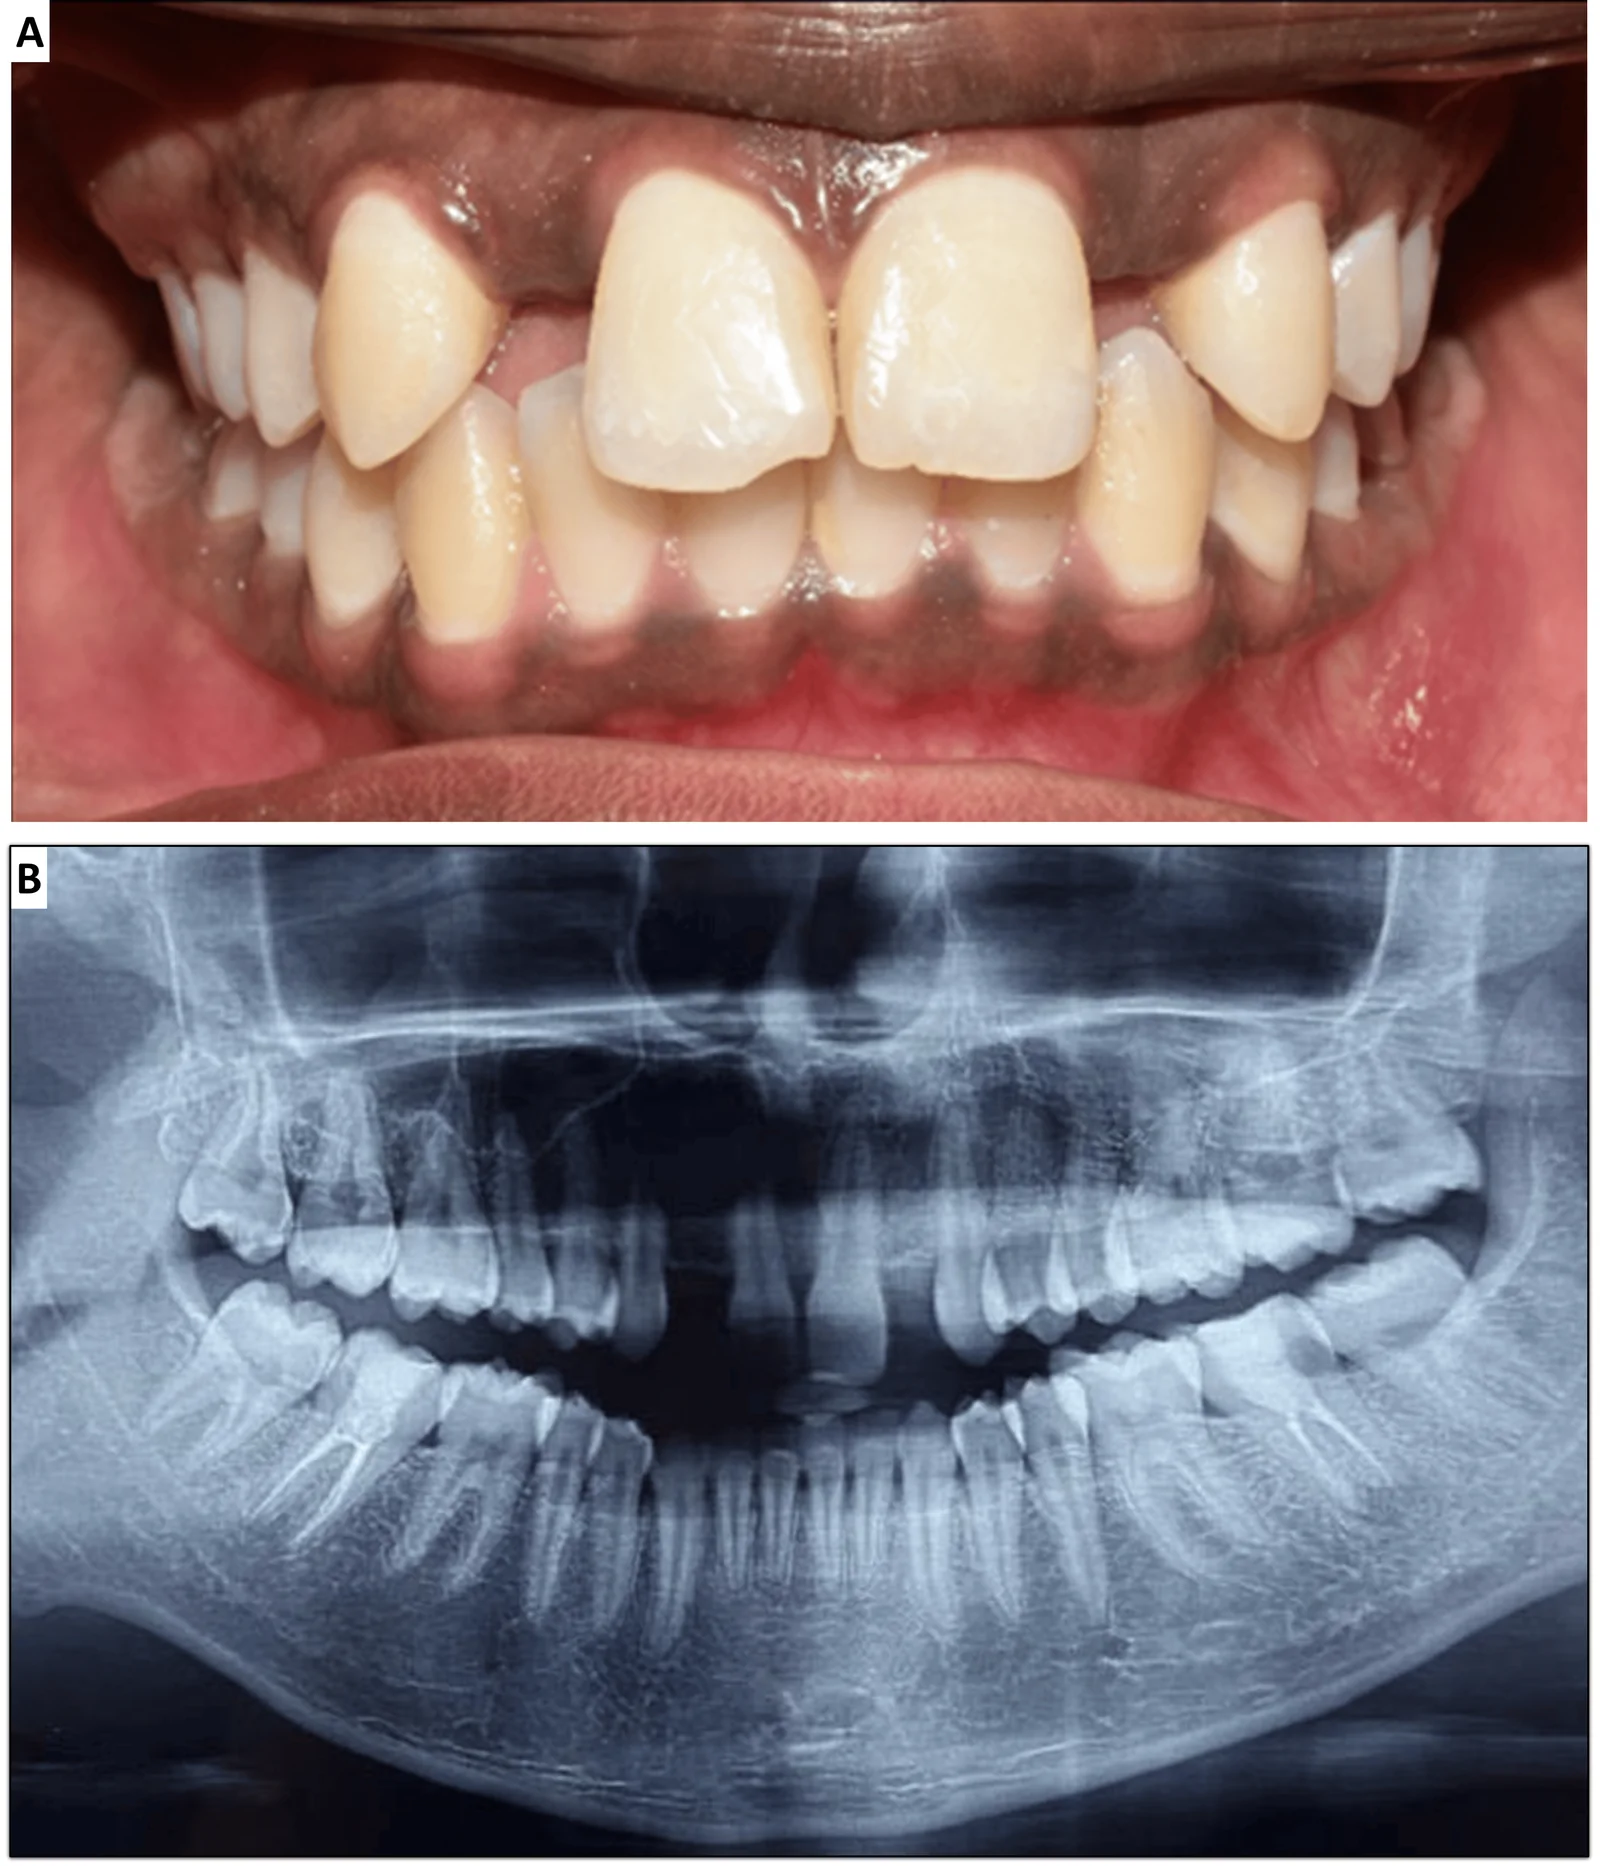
(A) Preoperative frontal intraoral view showing the missing maxillary lateral incisors and (B) orthopantomogram showing the healed extraction sites and supporting structures of the proposed abutment teeth
